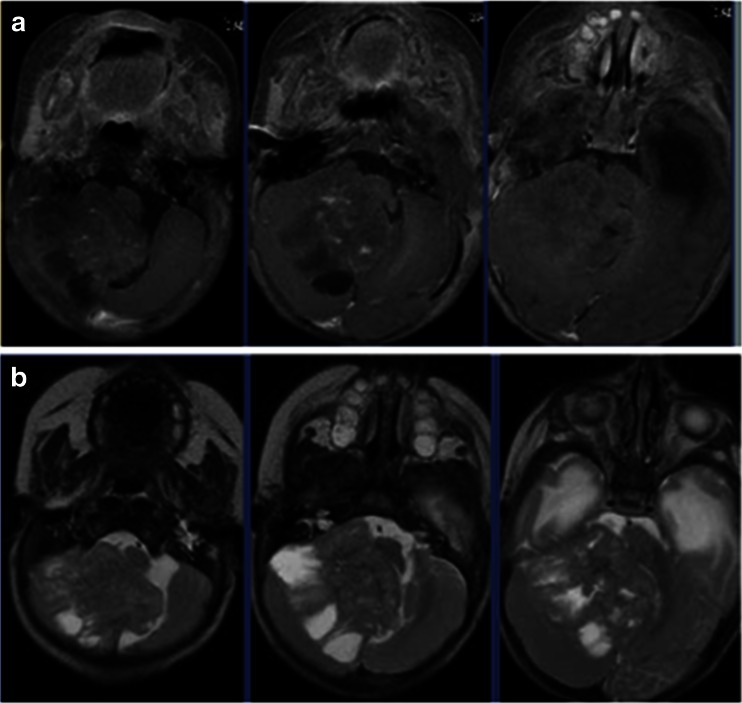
Case 1. Axial MR image (a T1-weighted post contrast, b T2-weighted) of a 3-month-old infant showing a large poorly enhancing, inhomogeneous right cerebellar ATRT extending into the CPA
